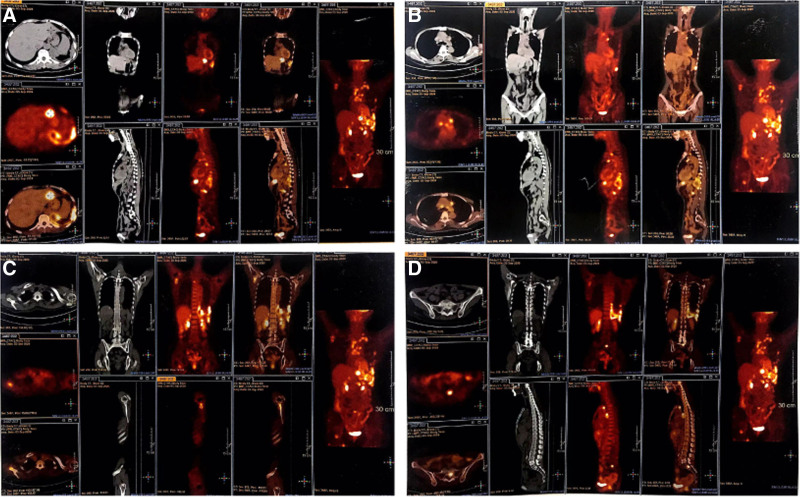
PET-CT high metabolic area. (A) Upper segment of left outer lobe of liver. (B) Multiple lymph node shadows on the left side of the abdominal aorta in mediastinum and retroperitoneum. (C) Left scapula. (D) Sacrum. PET-CT = positron emission tomography/computed tomography.
